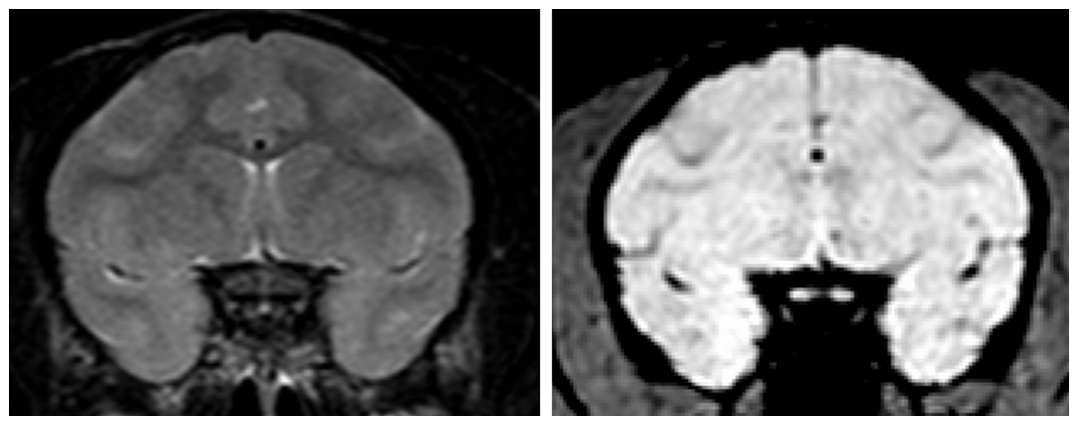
Example of T2-weighted (left) and SWI (right) MR images corresponding to the experiment from Figure 2. Edemas should appear brighter in T2-weighted images; hemorrhages, as well as large vessels should appear in black in SWI images. No damage was detected on any of the experiments performed.
